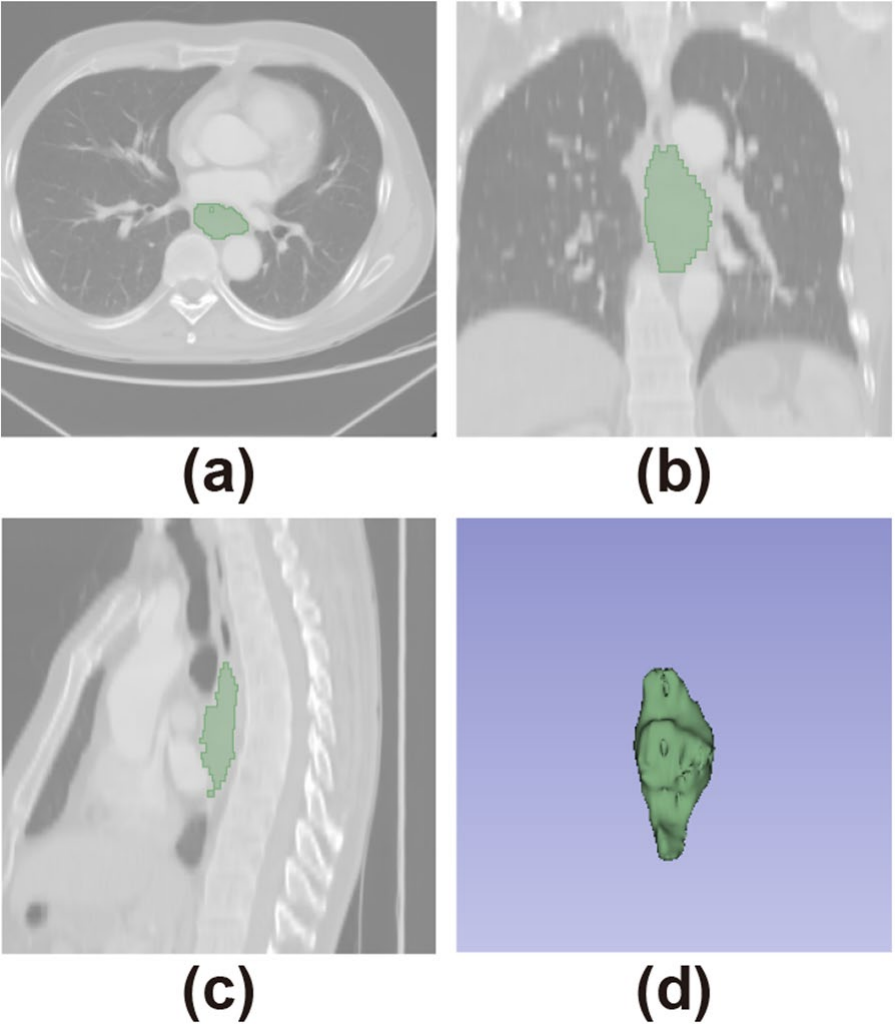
Sample ROI for CT images. a Segmentation on horizontal slices. b Segmentation on the coronal section. c Segmentation on sagittal slice. d 3D image of ROI
